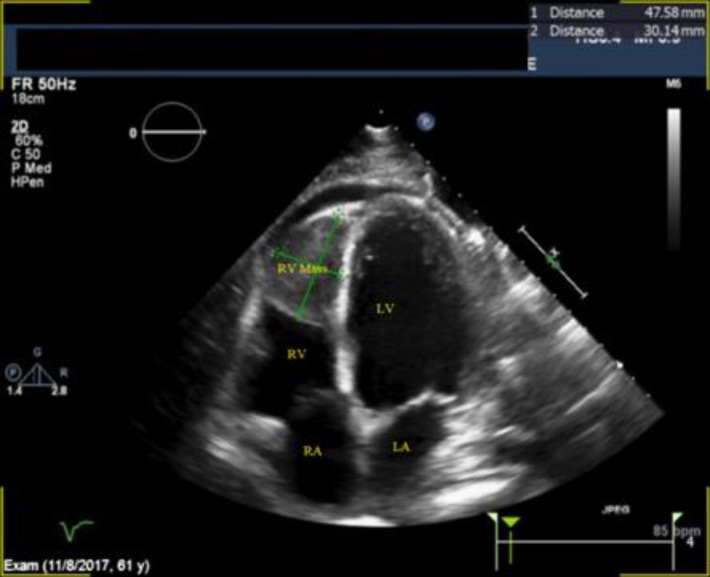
Apical 4-chamber view demonstrating a large mass measuring 4.8 cm x 3.0 cm extending from the apex to the mid right ventricular cavity. LA Left atrium; LV Left ventricle; RA Right atrium; RV Right ventricle
